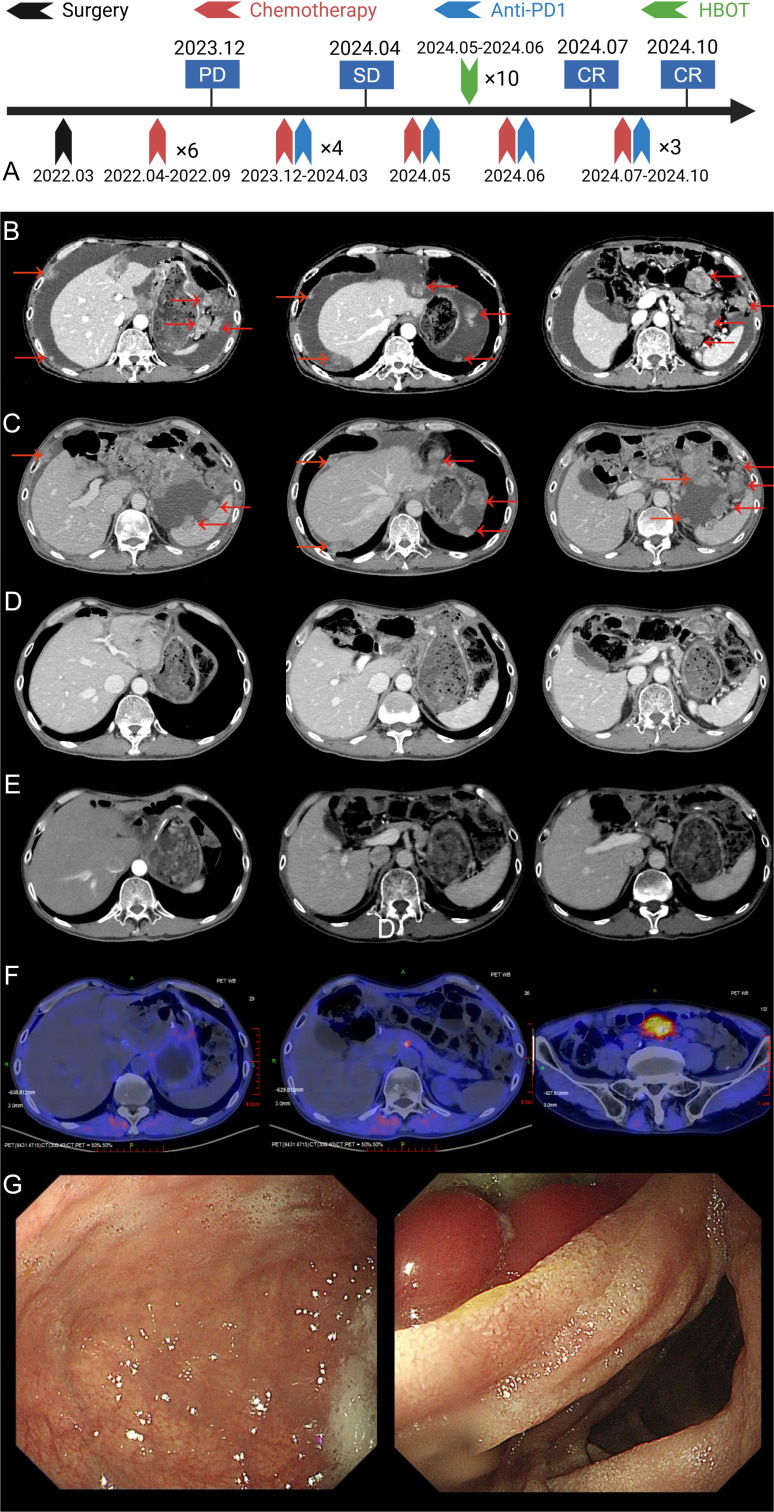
Imaging and treatment timeline of the patient. (A) The overall treatment timeline of the patient, including surgery, chemotherapy, anti-PD-1 therapy, and HBOT. (B) Abdominal enhanced CT at the time of recurrence showing tumor recurrence in the liver and surrounding tissues (tumor marked by red arrows). (C) Abdominal enhanced CT after 4 cycles of chemotherapy combined with ICIs treatment, showing partial tumor response (tumor marked by red arrows). (D) Abdominal enhanced CT after HBOT and an additional cycle of chemotherapy combined with ICIs, showing further tumor regression (tumor marked by red arrows). (E) Abdominal enhanced CT after 7–9 cycles of chemotherapy combined with ICIs, showing near-complete tumor resolution (tumor marked by red arrows). (F) FAPI PET/CT performed on January 3, 2025, revealing no evidence of tumor recurrence throughout the body. (G) Upper gastrointestinal endoscopy findings after 7–9 cycles of treatment. The gastric mucosa appeared smooth, and no active lesions were observed.
